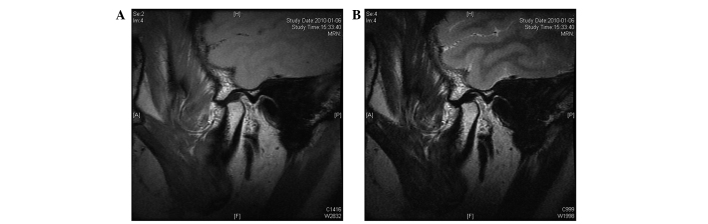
Preoperative magnetic resonance imaging (MRI) examination. Preoperative MRI reveals normal anatomic structures between the articular disk and the condyler in the (A) open and (B) closed jaw positions.
